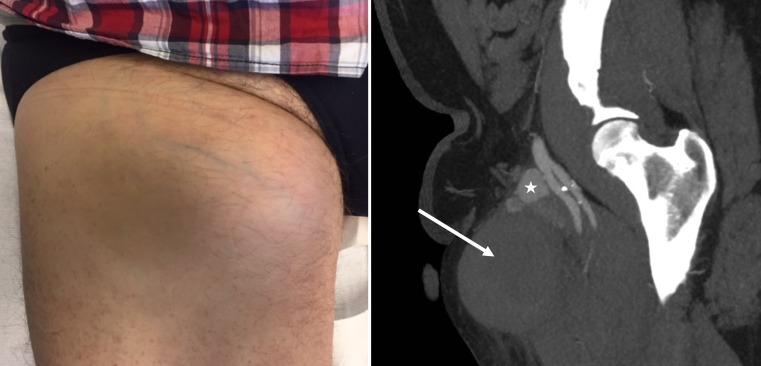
Clinical presentation of the large right groin mass (*left panel*). CT angiography demonstrating a pseudo-aneurysm of the femoral artery with an active component (*asterisk*), and a large haematoma anteriorly (*arrow*) (*right panel*)
